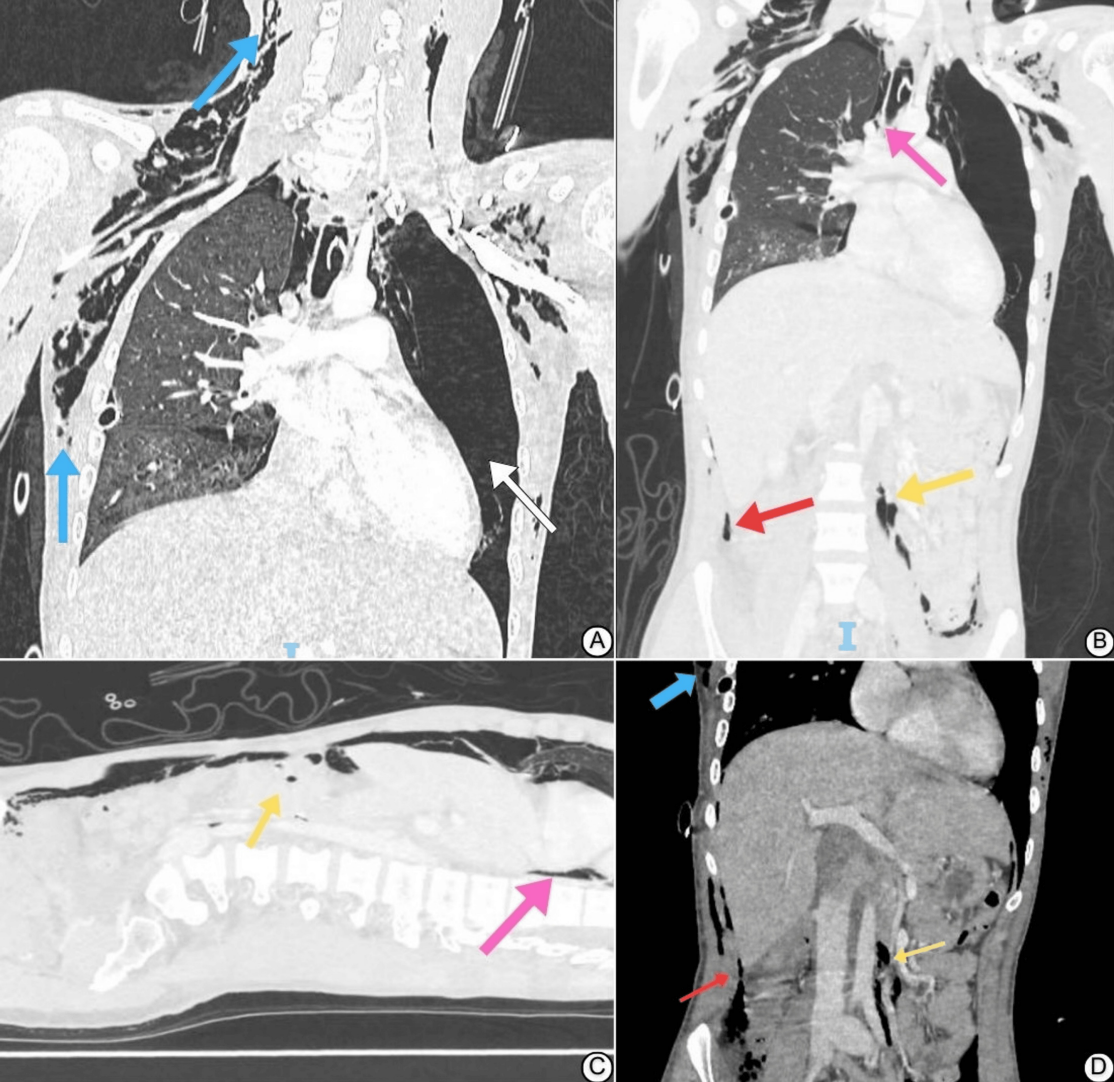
Coronal and sagittal computed tomography findings of the patient. (A) Coronal computed tomography chest view showing pneumothorax (white arrow) and extensive subcutaneous emphysema (blue arrows). (B) Coronal computed tomography chest view demonstrating pneumomediastinum (pink arrow) and pneumoperitoneum (red arrow). (C) Sagittal computed tomography view highlighting pneumoretroperitoneum (yellow arrow) and subcutaneous emphysema (blue arrow). (D) An abdominal window coronal computed tomography scan confirmed pneumoperitoneum (red arrow) and pneumoretroperitoneum (yellow arrow), with air tracking visible in the abdominal spaces, and previously noted subcutaneous emphysema (blue arrow).
